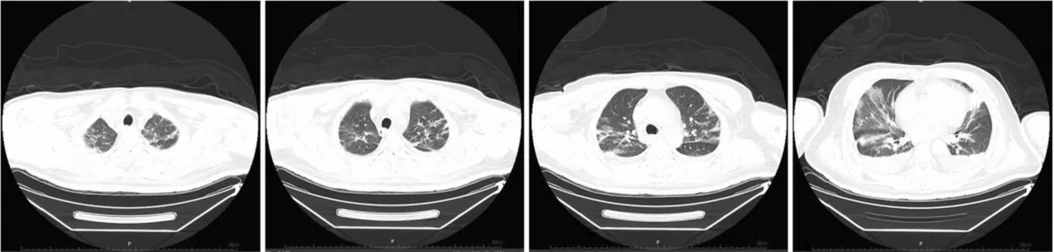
The chest CT of the patient on the eighth day of admission showed a double lung infection.
